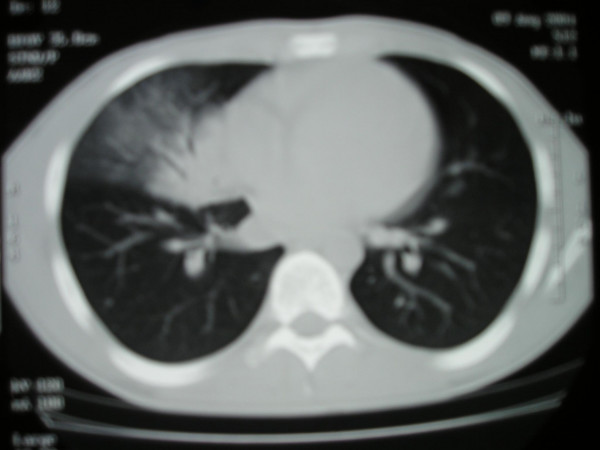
Computed tomography of the chest at admission showing consolidation with an air bronchogram in the right middle lobe and areas of atelectasis and ground glass opacities in the middle and lower right lobes.
